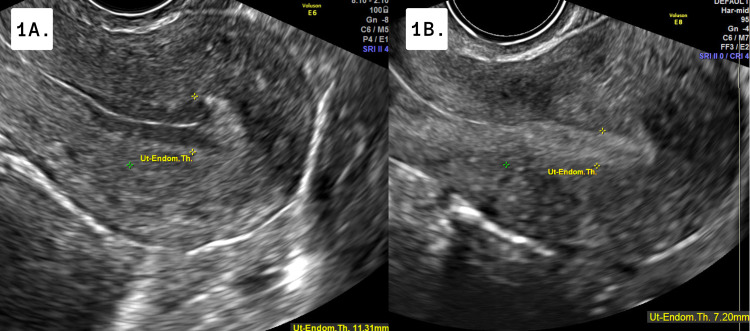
Transvaginal Ultrasound of Endometrial Lining 1A: Image of the adequately prepared endometrial lining of 11.31 mm after exogenous estradiol preparation at the initial sonogram appointment; 1B: Image of endometrial lining of 7.20 mm 7 days status post initial sonogram
